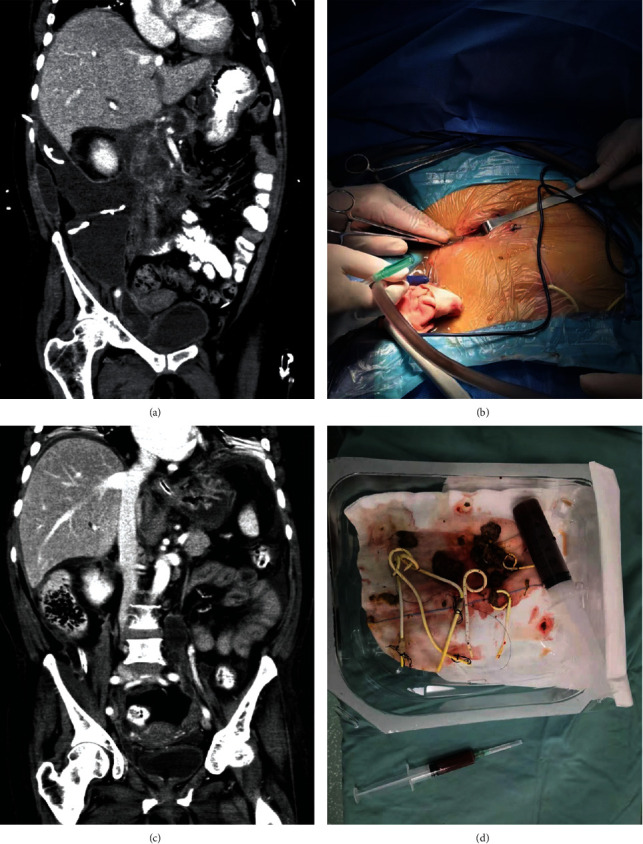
Small incision combined with nephroscope surgery for infectious pancreatic necrosis. (a) Preoperative CT showed a large amount of necrosis in the pancreas and intestinal space. (b) Incision of the transretroperitoneal approach surgery. (c) Postoperative CT showed that the necrosis was basically removed and absorbed. (d) Necrosis and exudates removed.
